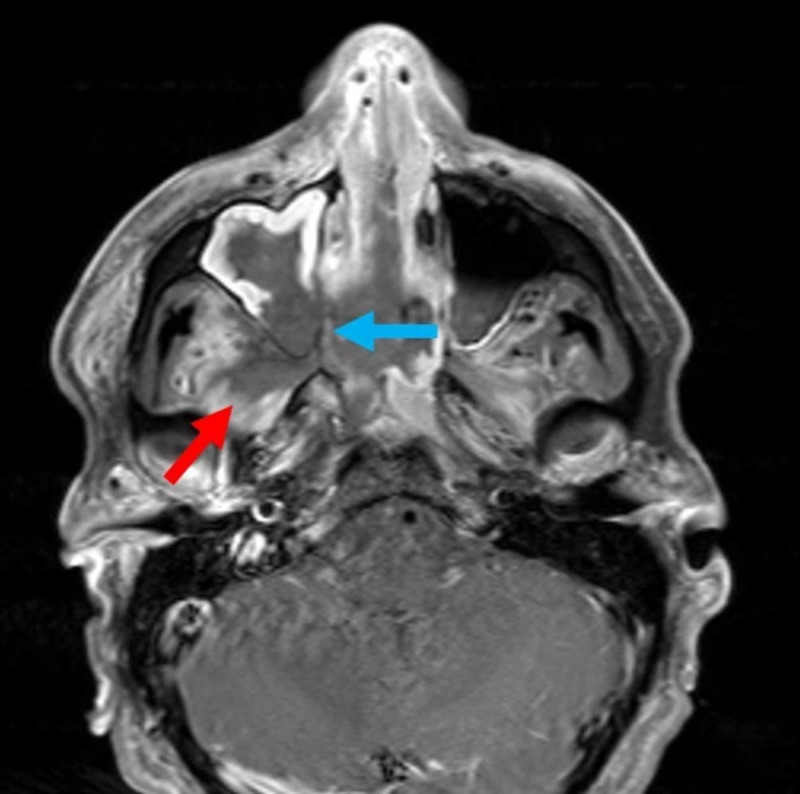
Axial T1 fat-saturated postcontrast MR image on day 2 of admission. There is marked enhancement of the bilateral pterygoid muscles indicating infection. The red arrow shows non-enhancing portion of the pterygoid muscles indicating necrosis. Worsening mucosal thickening and enhancement of both maxillary sinuses from spreading infection. The blue arrow shows non-enhancing mucosa of the right maxillary sinus indicating mucosal necrosis.
